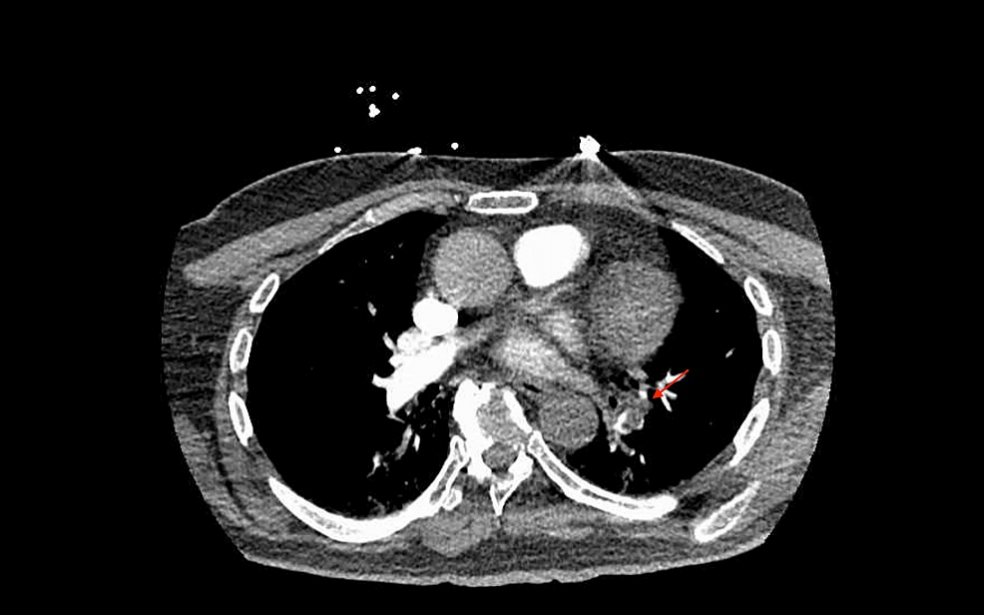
Computed tomography pulmonary angiography (CTA PE) showing left-sided segmental pulmonary embolus indicated with red arrow.
